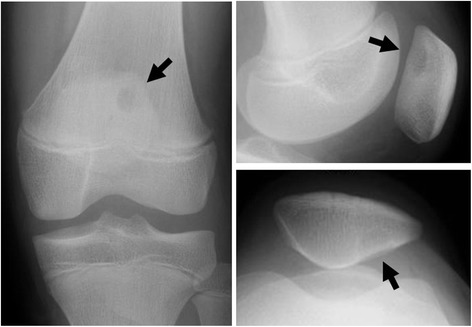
An 11-year-old girl with a dorsal defect of the patella. Plain radiographs show a well-defined osteolytic lesion with a sclerotic rim on the dorsal patella (black arrows)
